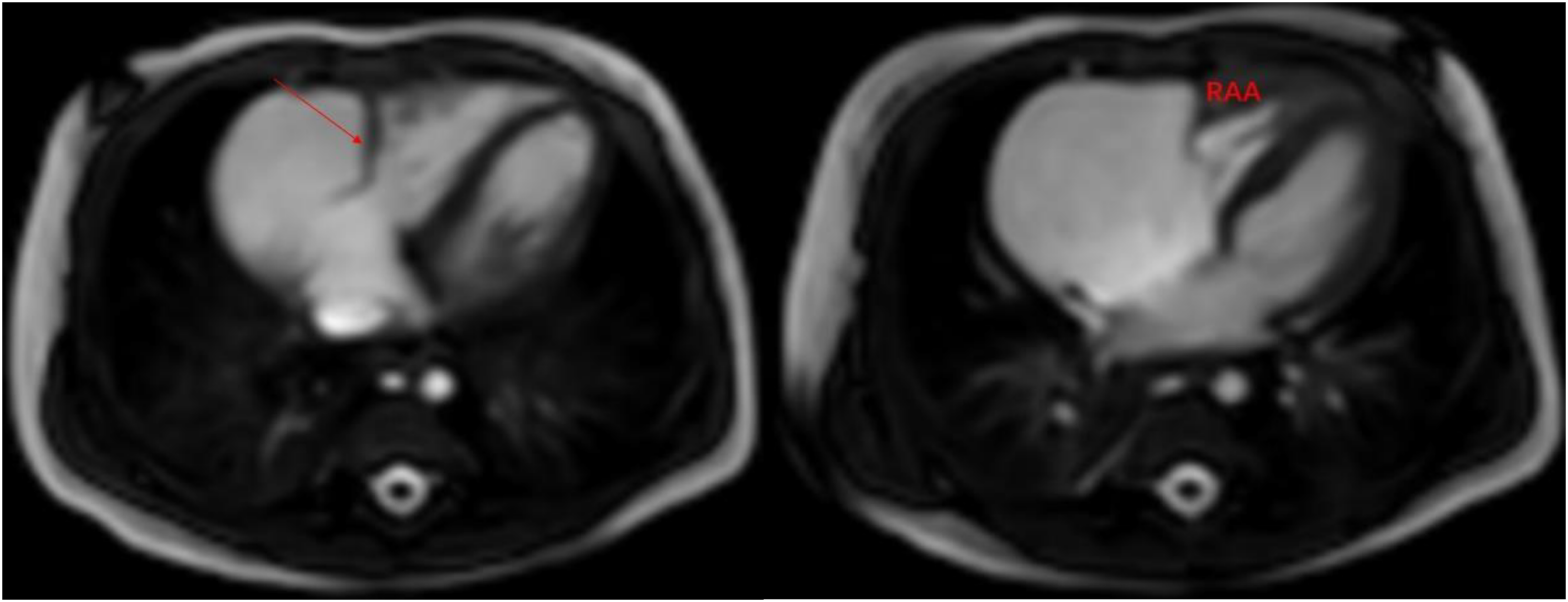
Cardiac magnetic resonance imaging: the arrow shows the atrial dissection. RAA, right atrial aneurysm.
